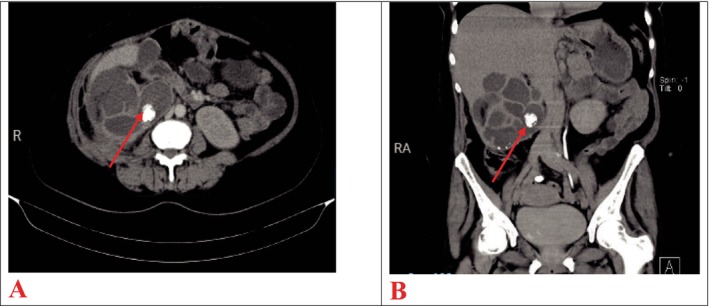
An axial (A) and coronal (B) CT images showing an enlarged, lobulated right kidney with low attenuating cystic spaces and hyperdense lesions (staghorn calculus) shown by the red arrow.
